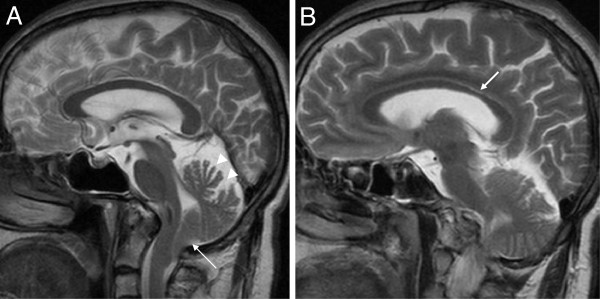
Developmental posterior fossa malformation resembling Chiari type I in the Thr458Ile+ p.Thr458Ile patient. (A) Midsagittal T2 MRI demonstrates herniation of cerebellar tonsils, resembling Chiari malformation type 1 (arrow). Also note the atrophy of the superior cerebellar vermis (arrow heads). (B) Para-midsagittal shows slight short-stretched thinning of the posterior mid-body of the corpus callosum (arrow).
